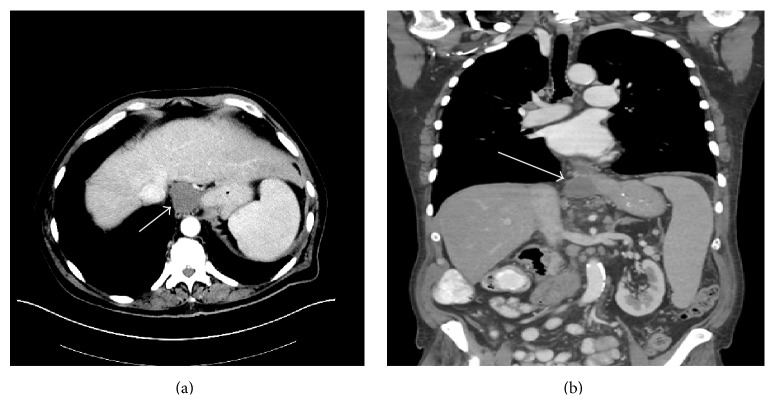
(a) Intravenous contrast enhanced axial CT scan of the abdomen demonstrates a well-circumscribed, low-attenuating, nonenhancing mass (arrow) adjacent to the gastroesophageal junction representing an enteric duplication cyst. (b) Coronal reformat of the intravenous contrast enhanced CT scan of the abdomen also demonstrates a well-circumscribed, low-attenuating, nonenhancing mass (arrow) adjacent to the gastroesophageal junction.
